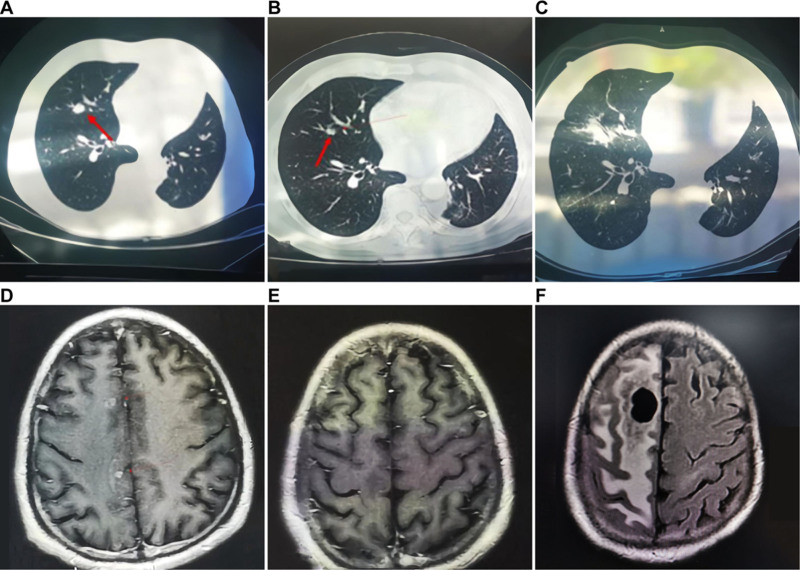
(A) Positive finding by CT scan before chemotherapy: nodules in the right upper lung lobe. (B) Positive finding by CT scan after chemotherapy: nodules in the right upper lung lobe. (C) CT scan after radiotherapy shows complete remission of lung nodules was found after 1.5 mo (D) Head MRI showed 2 brain metastatic lesions with 1 cm in diameter (E) Clinical response after gamma knife radiotherapy by head MR showed no brain metastasis. (F) Head MRI showed giant metastasis around the OMMAYA sac. CT = computerized tomography, MRI = magnetic resonance imaging.
